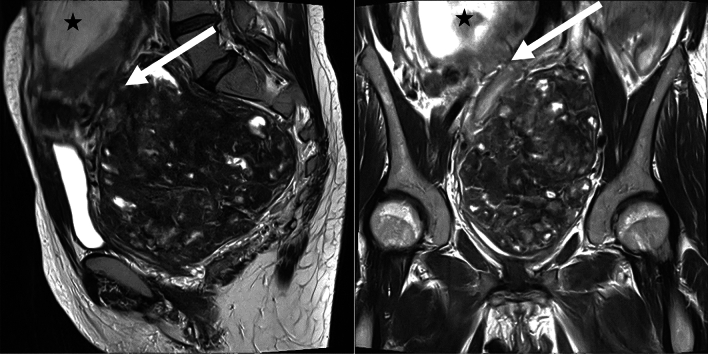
Preoperative MRI. T2-weighted images in the sagittal and coronal plane depicts a pedunculated sub serosal Leiomyoma (arrowhead) and the fetus (asterisk). The vascular pedicle visualized by worm-like signal-free vessels (arrowhead) suggests an attachment side of the leiomyoma to the posterior wall of the uterus. Degenerative changes (white spots) are seen within the leiomyoma
